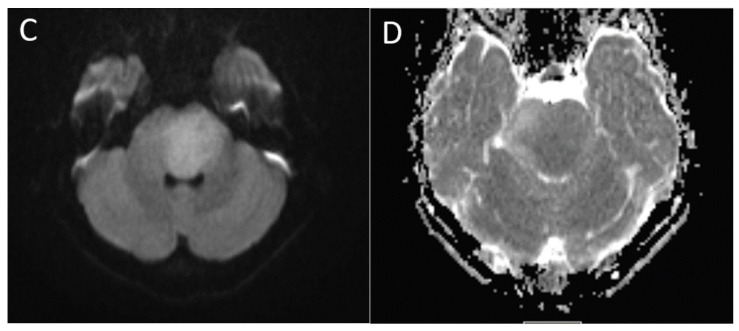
A 5-year-old boy with diffuse midline glioma H3K27-altered. Axial T2 (A), post-contrast T1 (B) images demonstrate a poorly marginated infiltrative, expansile T2 hyperintense lesion centered in the pons with little to no enhancement. DWI (C) and ADC (D) images demonstrate heterogenous signal without significant diffusion restriction.
